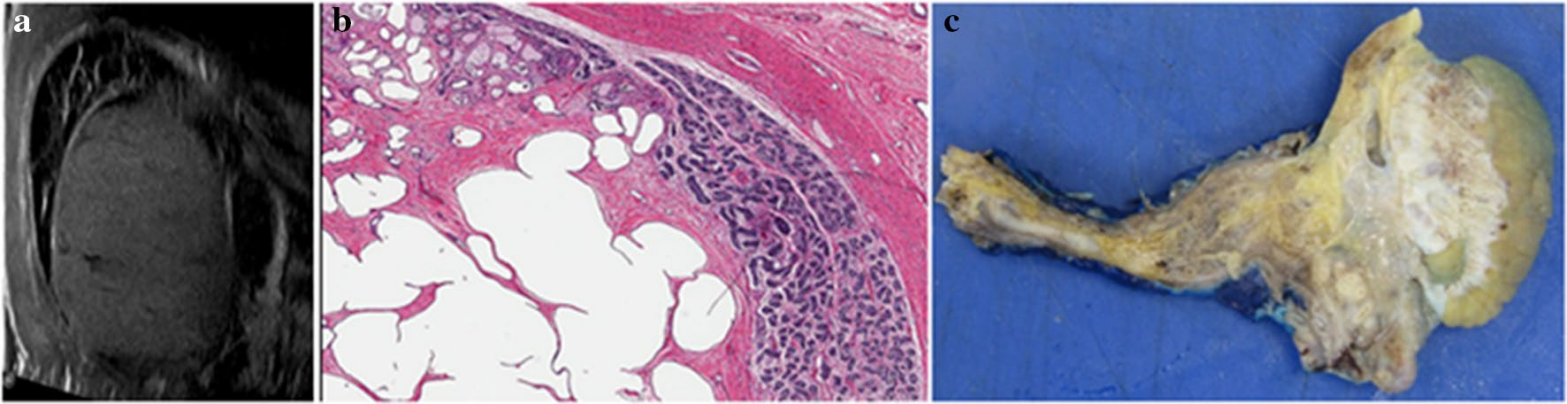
Large complex left para-testicular mass in 14-year-old male. a Ultrasound: distortion of the upper pole with a focal hypoechoic lesion with peripheral blood flow. Adjacent, but separate to this mass is an ill-defined heterogeneous area of abnormality which appears infiltrative into the normal parenchyma. b Intra-operative frozen section confirmed benign pathology. Benign multi-cystic lesion comprising variable sized cysts compressing the native testis and mediastinal rete testis. c Pathology—cystic dysplasia of the rete testis. Orchidectomy was performed as the lesion was so large and rest of the testicular tissue was atrophic. Final pathology: cystic dysplasia of the rete testis
